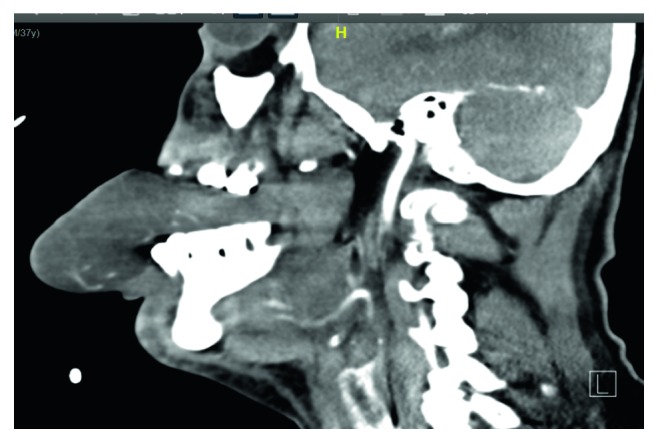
Sagittal cross section of a soft-tissue window computed tomography image with a tongue protrusion far beyond the lower lip.
